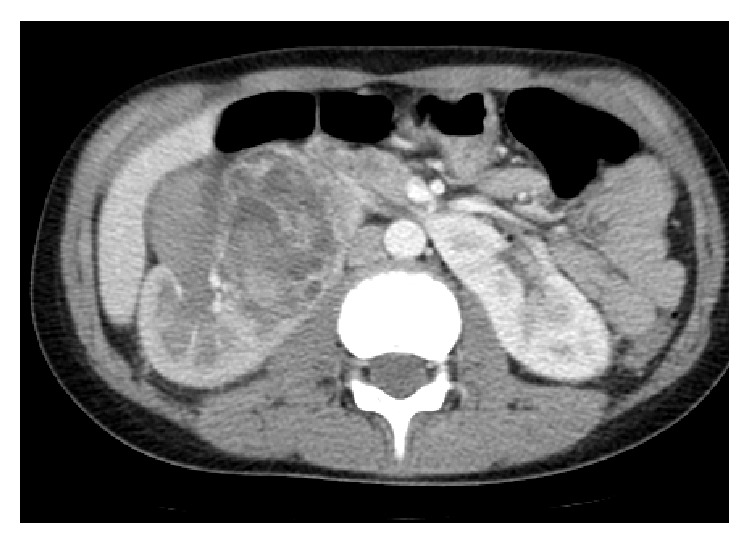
Abdominal computed tomography revealed an ill-defined tumor arising from the lower pole of the right kidney.
